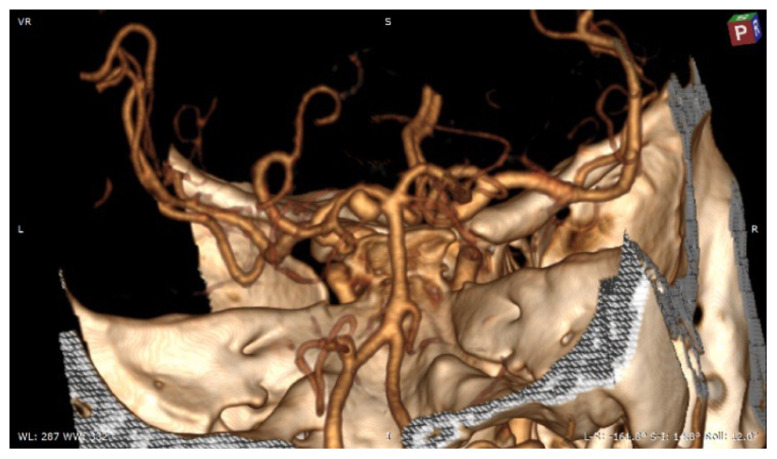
CTA 3D reconstruction shows proximal left SCA aneurysm measuring 4.8 × 6.7 mm
